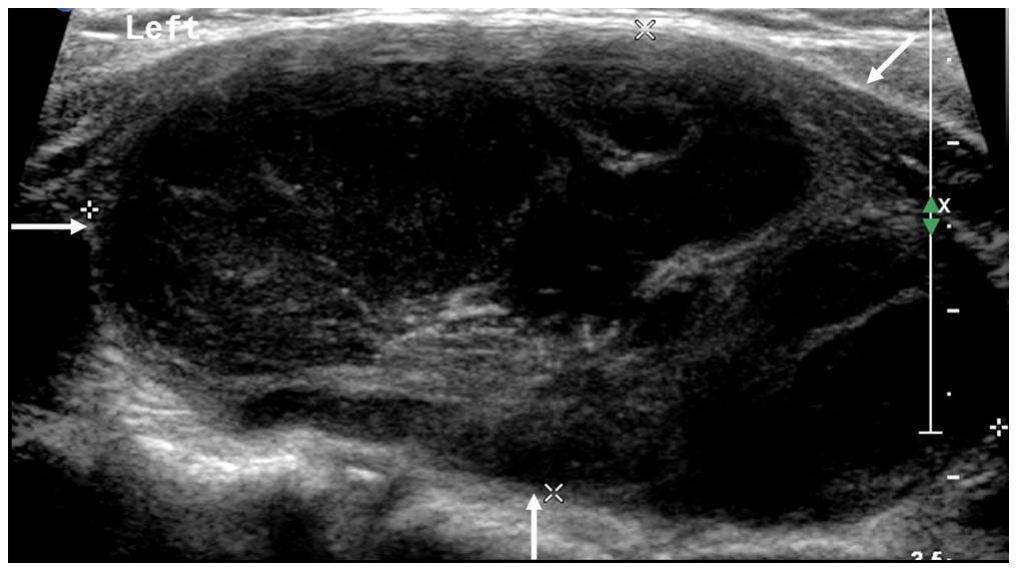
A 71-year-old female patient with diffuse PTL. The longitudinal sonogram of left lobe shows the marked hypoechogenecity with posterior acoustic enhancement (arrows).
